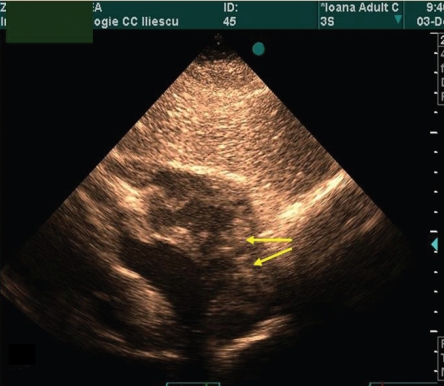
Subcostal apical four–chamber view. The right atrium is the anterior cavity. The arrows point at the multiple sites of tumor insertions in the right atrial wall.
